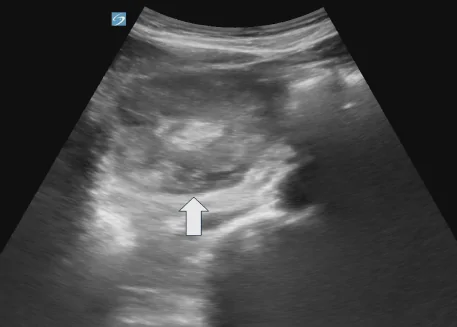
Point-of-care ultrasound (transverse view, right mid-abdomen) demonstrating the classic “target” sign consistent with ileocolic intussusception. Concentric hypoechoic and hyperechoic rings represent the bowel-within-bowel configuration.
